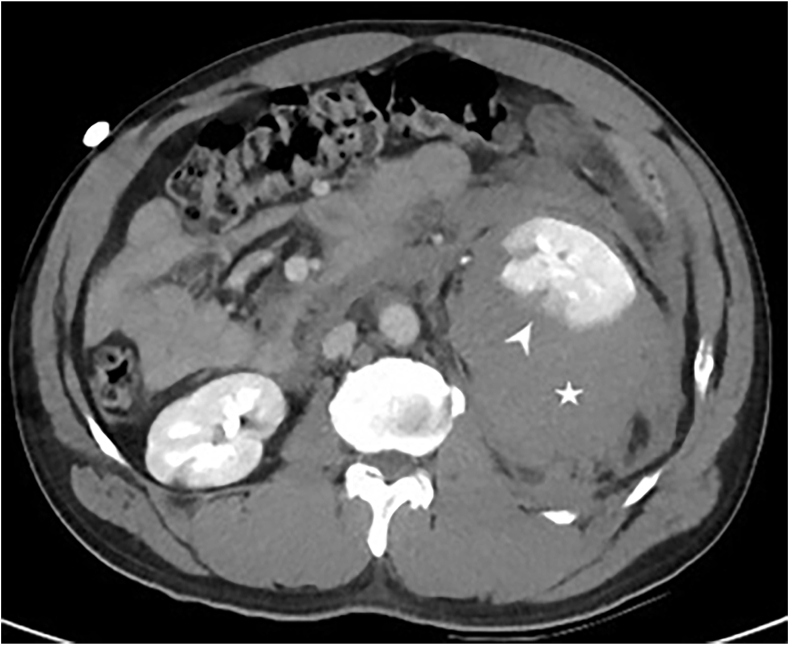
The initial contrast enhanced CT showed a large peri-renal hematoma at the left kidney (star), and a laceration at the lower pole of the kidney (arrow).
